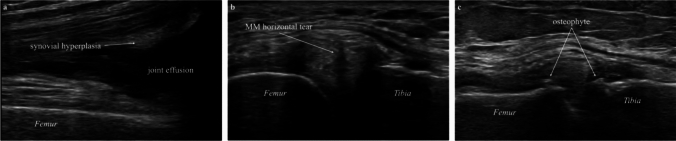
Ultrasonographic findings of synovial hyperplasia, joint effusion, horizontal tear of the medial meniscus, and osteophytes of the medial condyle of the femur and tibia Synovial hyperplasia and joint effusion were observed in the suprapatellar bursa (a). The largest portion of the synovial thickened area and the joint effusion was measured, and a diameter ≥4 mm was considered positive. Horizontal tears of the medial meniscus and osteophytes were observed on the long-axis image, in which the medial collateral ligament was most clearly depicted. A horizontal tear of the medial meniscus was considered positive if there was a hypoechoic area in the meniscal parenchyma (b). Osteophytes were identified bilaterally on the femoral and tibial sides. In the presence of osteophytes, bony prominences were identified at the site of the articular cleft, and in the case of cartilaginous osteophytes, a double overlap of hyperechoic areas was identified (c)
